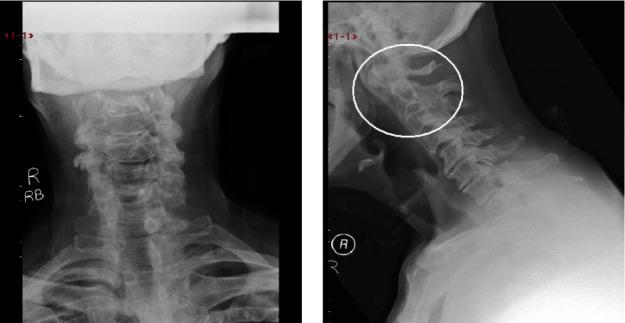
Plain x-rays of the cervical spine revealing spondylosis and increase in the distance between anterior atlas and odontoid peg
